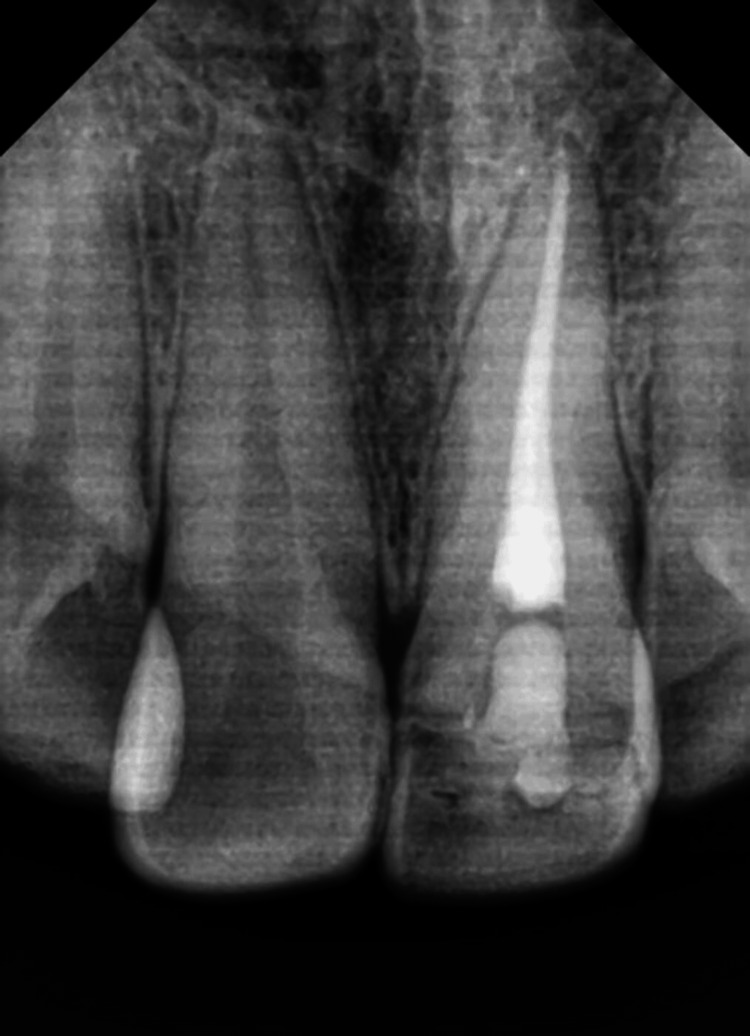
Postoperative radiograph with grooves made in the fragment
